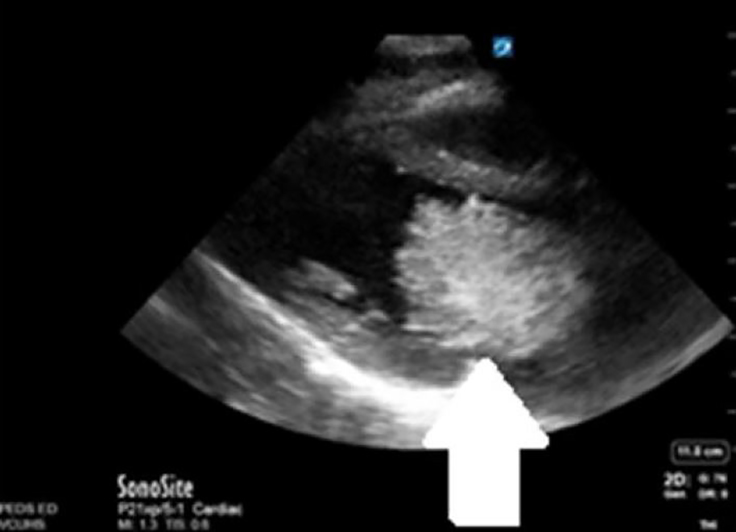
Parasternal long-axis view of left atrial myxoma (arrow).
